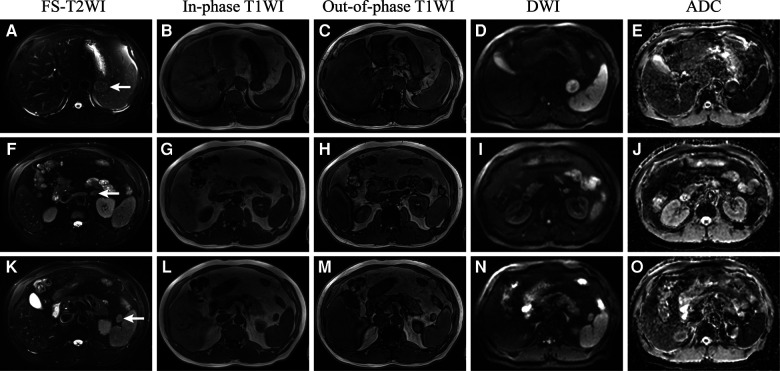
Retroperitoneal ectopic pancreas (A–E), adrenal adenoma (F–J), and accessory spleen (K–O) in FS-T2WI (fat suppressed T2WI), in-phase T1WI, out-of-phase T1WI, diffusion-weighted imaging (DWI), and apparent diffusion coefficient (ADC).
